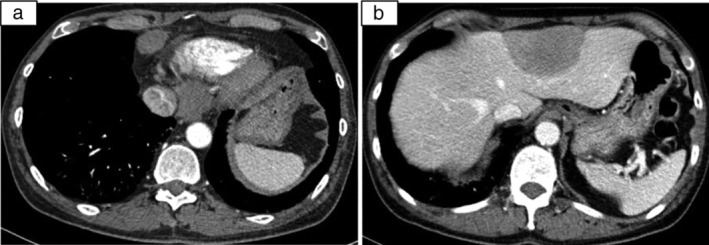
Contrast chest and abdominal CT showed enlarged mediastinal and retroperitoneal lymphadenopathy (a) and a huge left hepatic mass (b).
